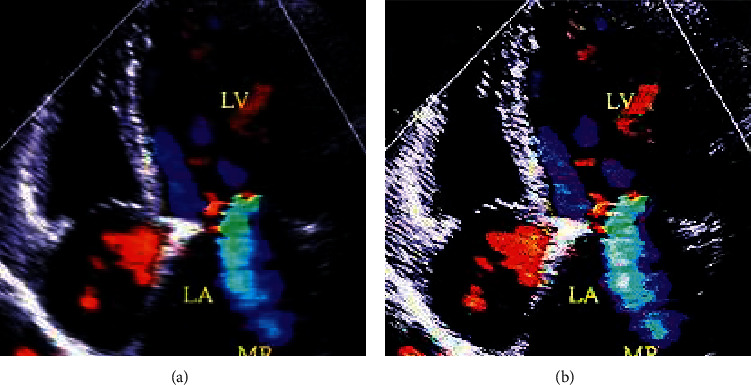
Comparison on cardiac color ultrasound images of CHF patients between the two groups. (a) Control group; (b) experimental group.
